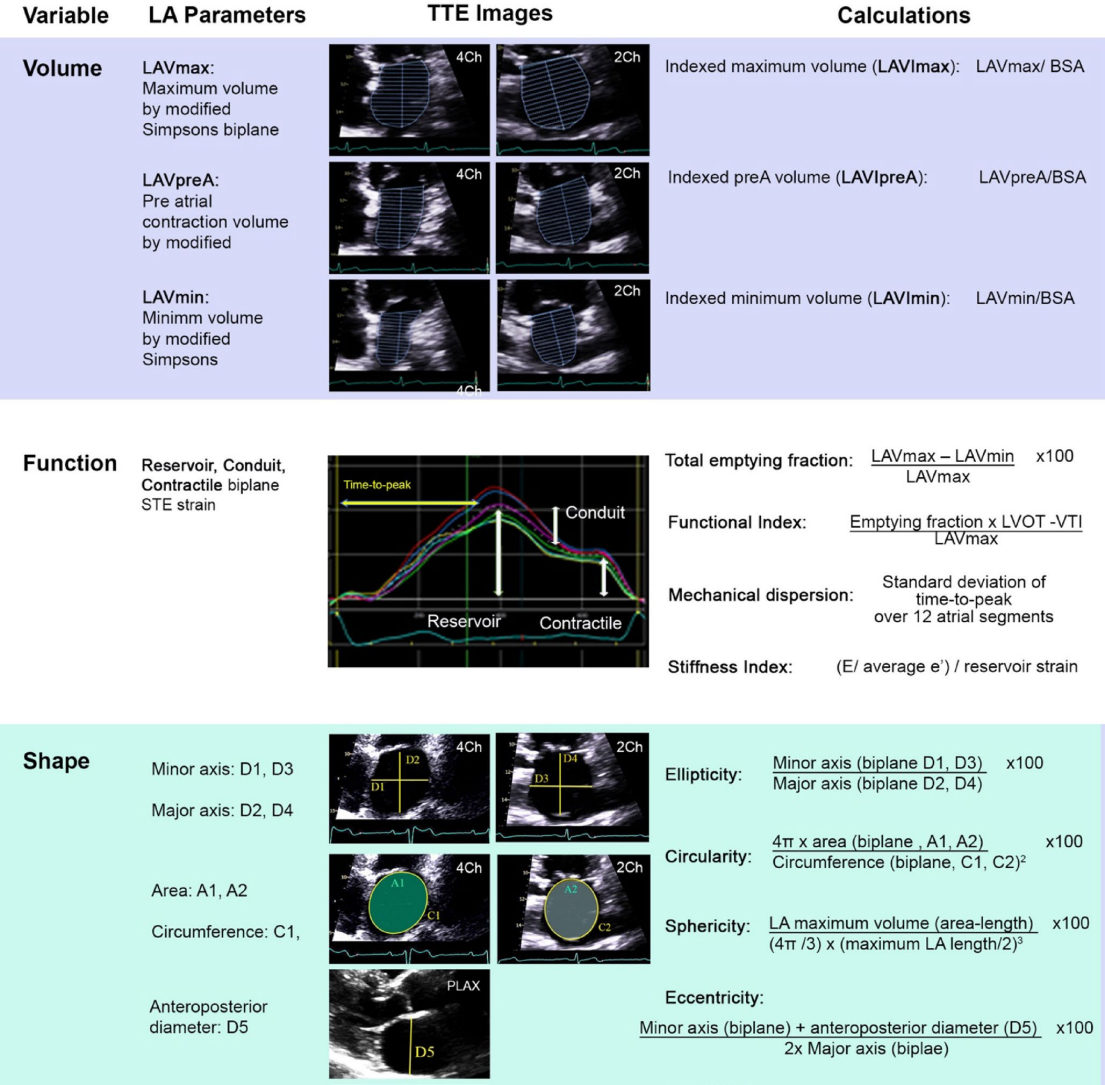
Comprehensive transthoracic echocardiogram assessment of left atrial volume, function, and shape. BSA: body surface area. LA: left atrial. TTE: transthoracic echocardiogram
